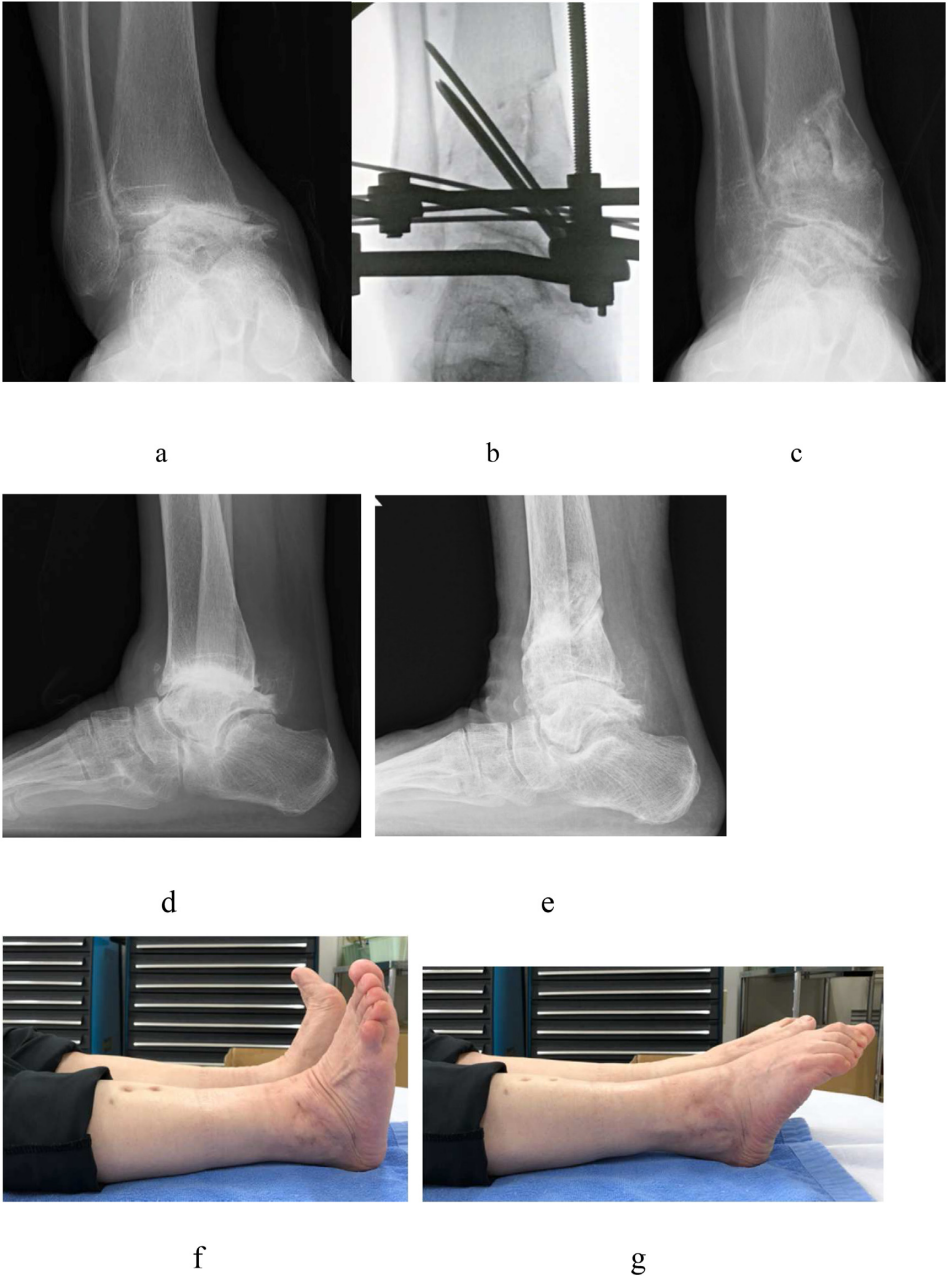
A 75-year-old female. Right ankle osteoarthritis, stage 3b (case 3). Severe varus deformity is observed in this case with an excessive talar tilt. The point different from case 2 is the talar medial shift. Although the talar tilt remains after distal tibial oblique osteotomy (DTOO), the clinical results improve markedly. a. Preoperative weight-bearing ankle radiograph (AP view). Severe medial shift and tilt of the talus are observed, and the articular surface of the medial malleoli and medial tibial plafond is caving in. b. Intraoperative ankle fluoroscopy (AP view). DTOO was performed with elongation of the Achilles tendon and resection of bony spurs. c. Postoperative weight-bearing ankle radiograph (AP view). The medial shift and tilt of the talus were corrected after DTOO, but the incongruity of the tibial plafond and talar dome seemed to remain. d. Preoperative weight-bearing ankle radiograph (lateral view). The talus seems to be flattened, and the joint space is unclear. e. Postoperative weight-bearing ankle radiograph (lateral view). The joint surface seems to be clear after DTOO. f. Postoperative ankle dorsiflexion appearance. Dorsiflexion of the ankle joint in knee extension position is 0°. g. Postoperative ankle plantar flexion appearance. Plantar flexion of the ankle joint in knee extension position is 35°.
